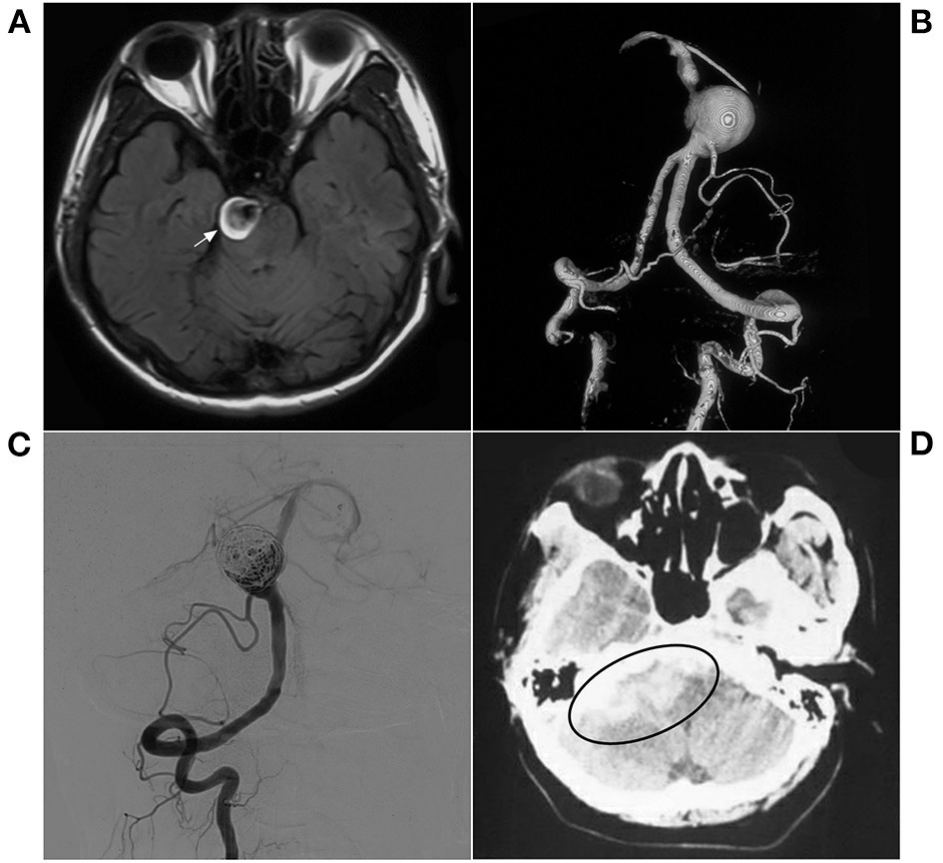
EVT with a delayed hemorrhage. (A) MR T2WI shows an aneurysm in the right pons (arrow); (B) 3D-reconstructed DSA shows a spherical aneurysm in the inferior BA. (C) Postoperative VA-DSA shows the aneurysm following simple coiling embolization, MRRC Grade IIIa. (D) 1-month postoperative CT shows hemorrhage in the lateral brain stem (ellipse). BA, basilar artery; CT, computerized tomography; DSA, digital subtraction angiography; EVT, endovascular therapy; MR, magnetic resonance; MRRC, Modified Raymond-Roy Classification; VA, vertebral artery.
